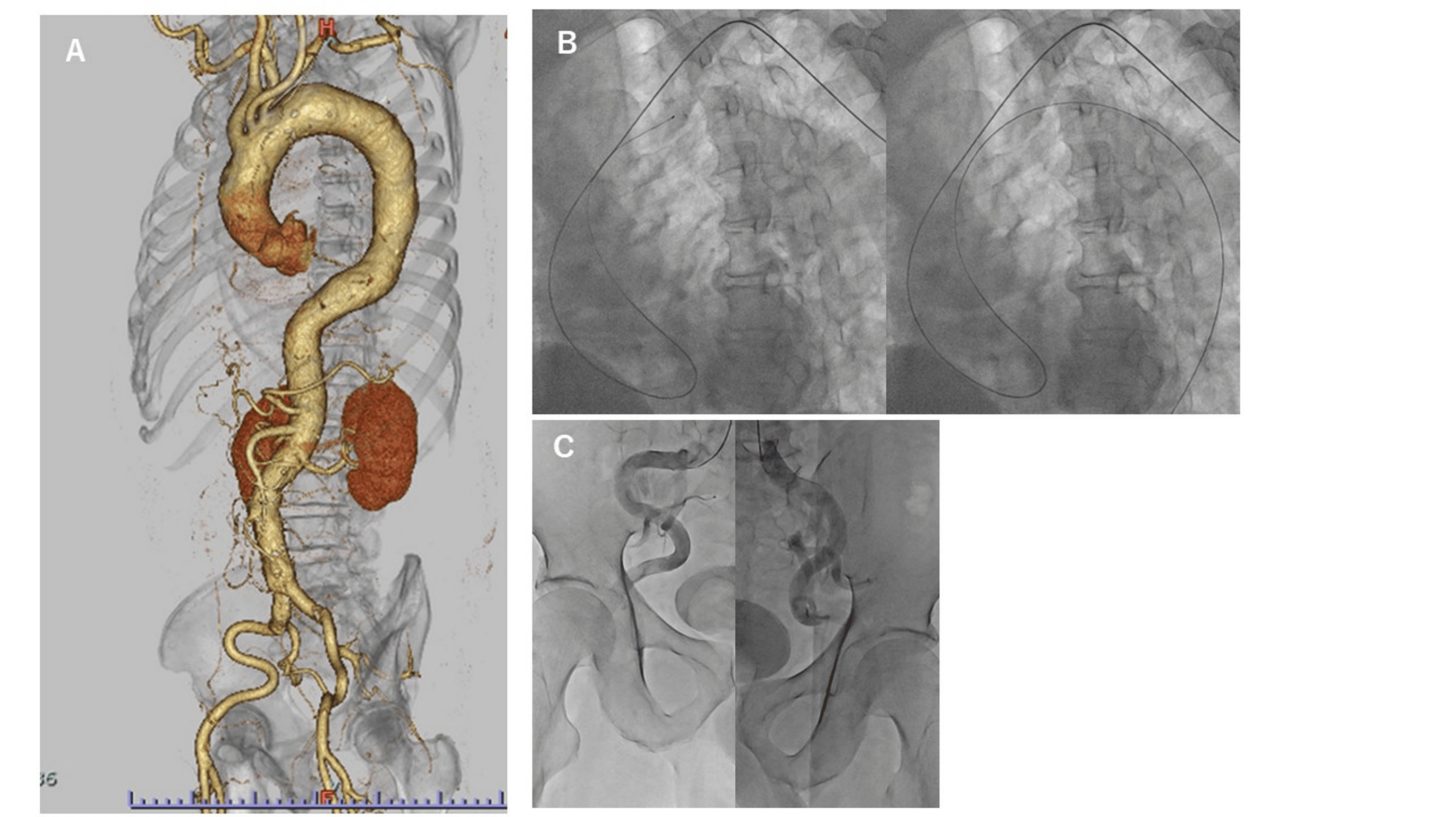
Lower limb angiography in Case 3. (A) The aortic arch is type III on contrast-enhanced computed tomography. The descending aorta is also highly curved. (B) The catheter was advanced in the same way as in case 2, but when it was pulled up and straightened, it bent again when pushed in again. (C) The tip of the catheter could only be advanced to the bilateral common iliac arteries.
